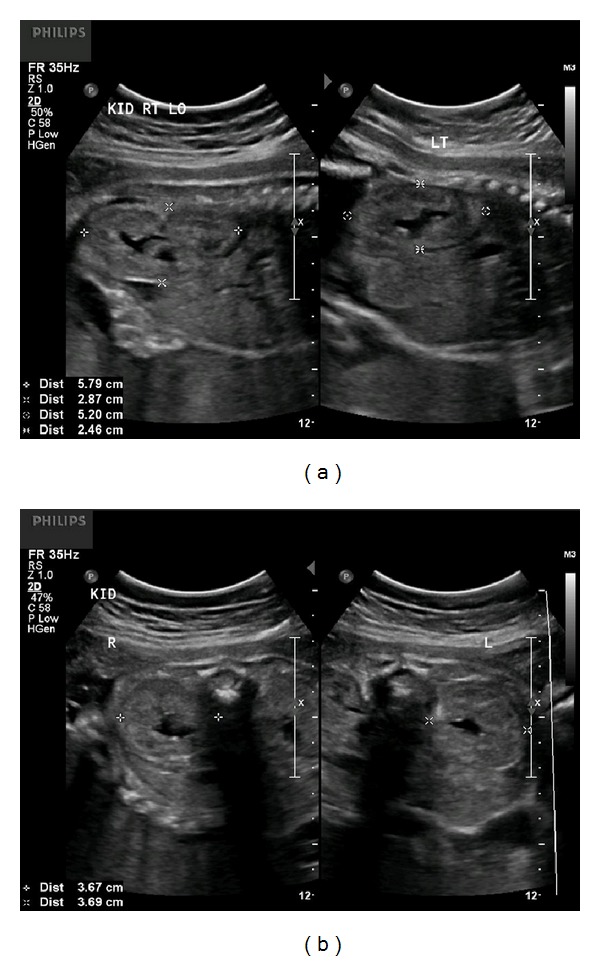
(a) Longitudinal images of the fetal kidneys at 29 weeks and 1 day of gestation. The kidneys continue to be enlarged and echogenic in appearance. Oligohydramnios was noted at the time of this ultrasound. (b) Transverse images of the fetal kidneys at 29 weeks and 1 day of gestation.
